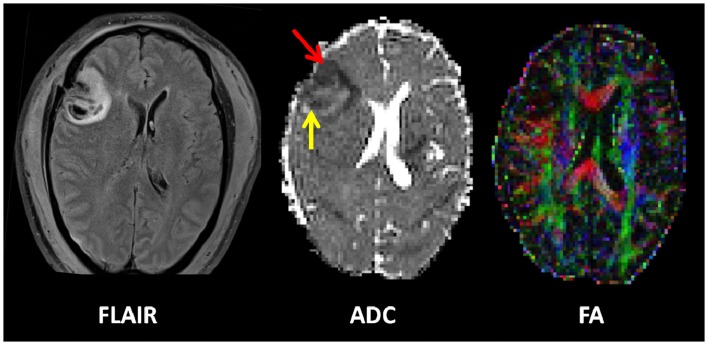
Example of an early contusion in a 49-year-old male who sustained a severe TBI after an alleged assault. He was GCS 4 at the scene. Imaging was performed approximately 48 h after injury. A left frontal contusion can be clearly seen on the FLAIR image. The apparent diffusion coefficient map (ADC) shows a cytotoxic rim (red arrow) and vasogenic rim (yellow arrow). The combined fractional anisotropy and directional map (FA) shows loss of fibers integrity at the site of the contusion. Color hue indicates direction as follows; red, left–right; green, anteroposterior; blue, superior–inferior.
